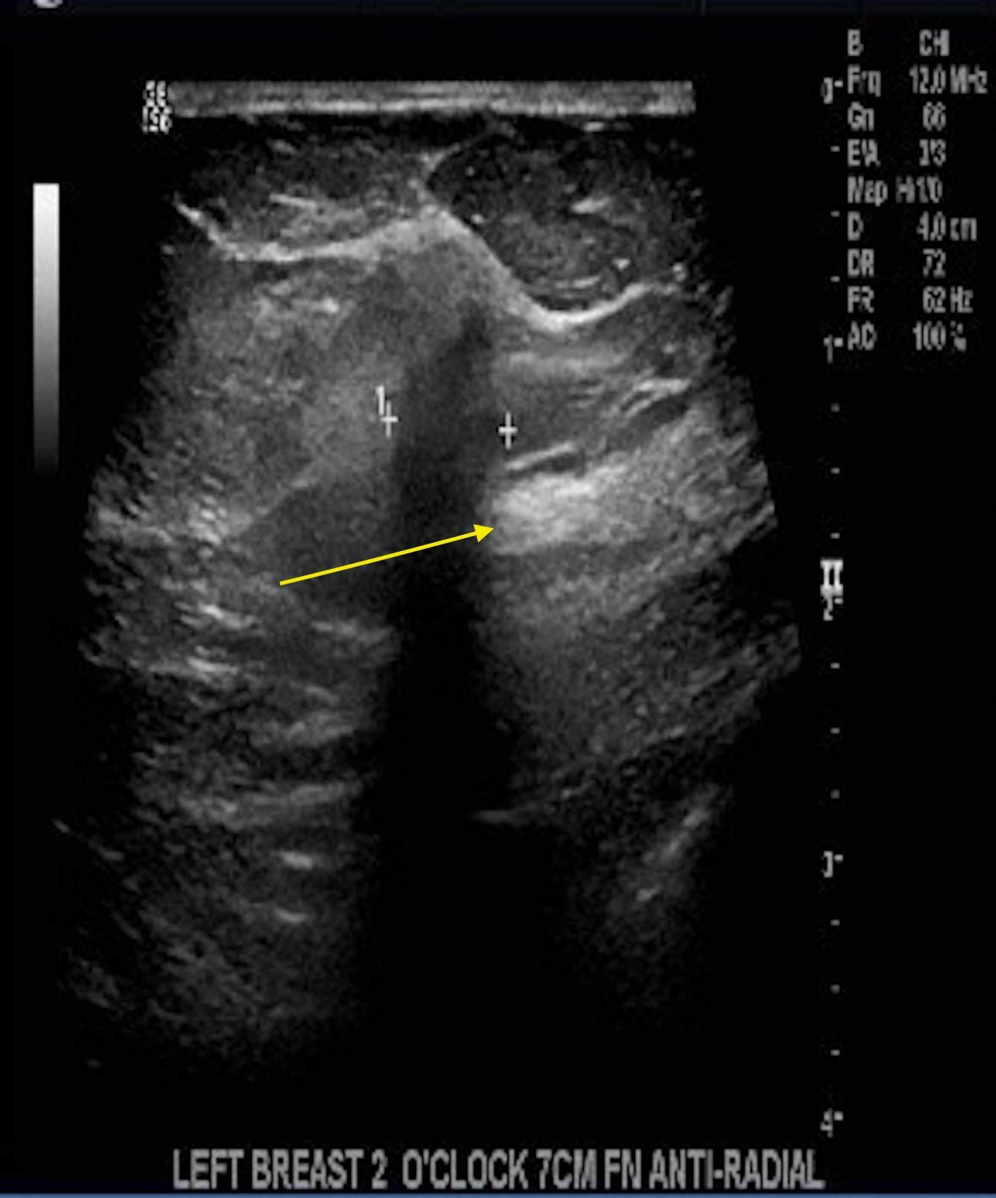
Ultrasound of the left breast A 4-mm hypoechoic nodule at the 3’o clock position, 4 cm from the nipple, can be seen.
